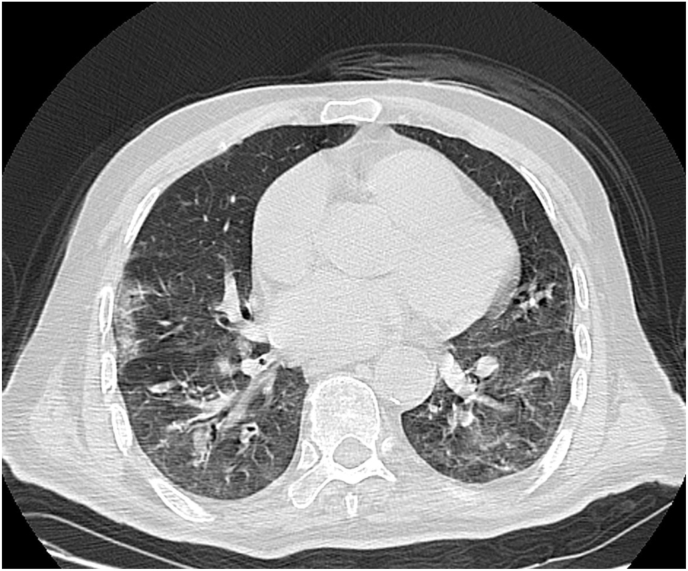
Axial image (lung windows) from the CT pulmonary angiogram demonstrating extensive multifocal patchy ground-glass opacities in lung parenchyma.
